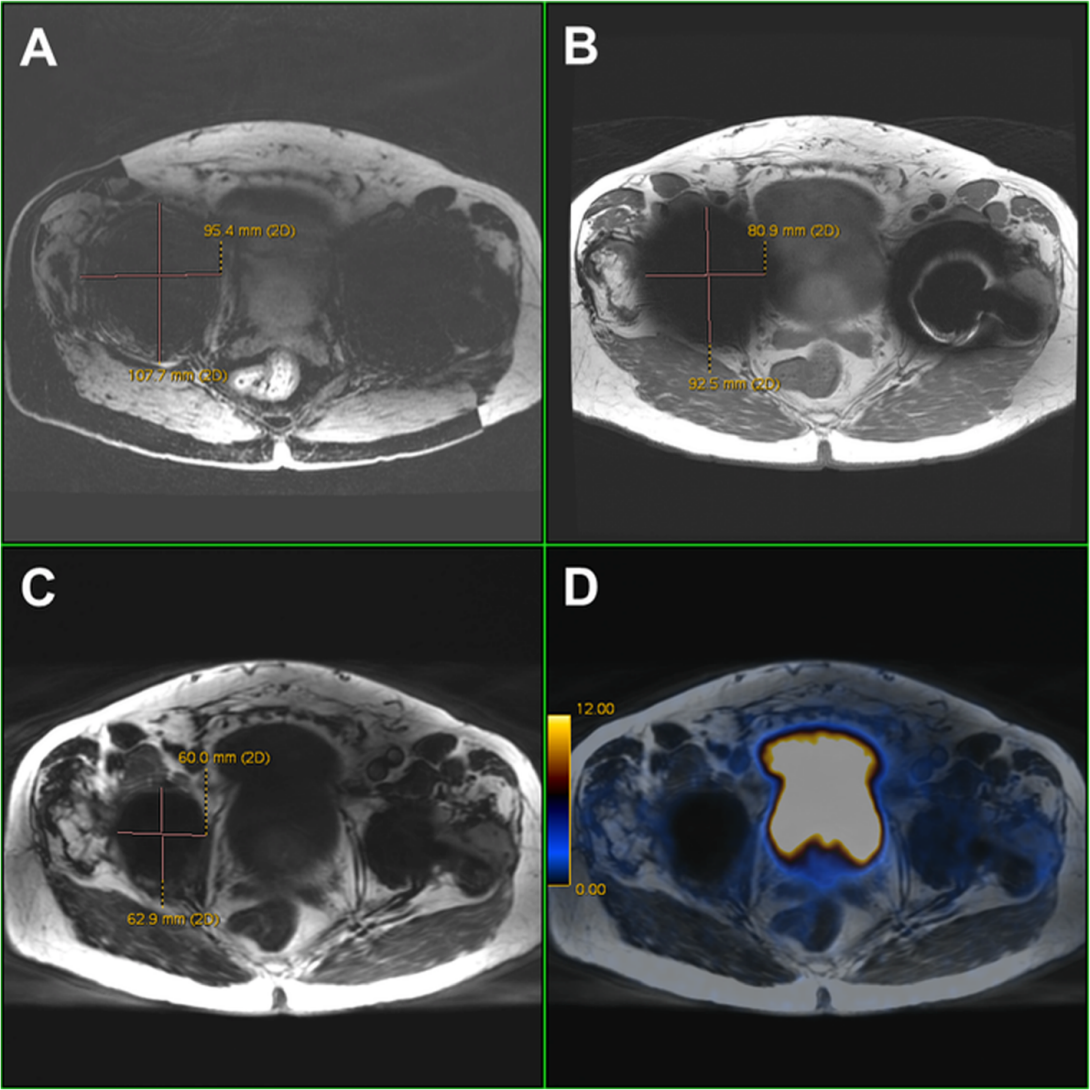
Different appearances of MR signal voids due to hip joint implants. Axial images of a patient with bilateral hip joint implants, referred to ^68^Ga-PSMA-11 PET/MRI for staging prostate cancer. a LAVA-FLEX. b T1w-FSE. c MAVRIC-SL show the different size of signal voids, with the corresponding fused image. d PET/MRI MAVRIC-SL
